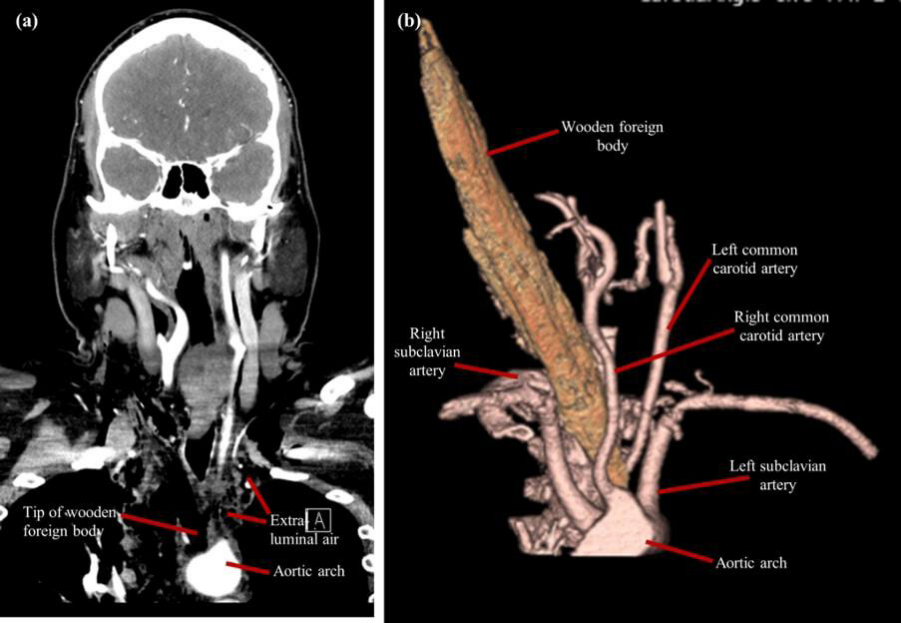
(a and b) An anterolateral CTA with 3D volume rendering showing the presence of the wooden foreign body amongst the major vessels originating from the aortic arch, with an apparent absence of the brachiocephalic trunk. The aberrant right subclavian artery is visualised travelling retropharyngeal, posterior to the foreign body.
